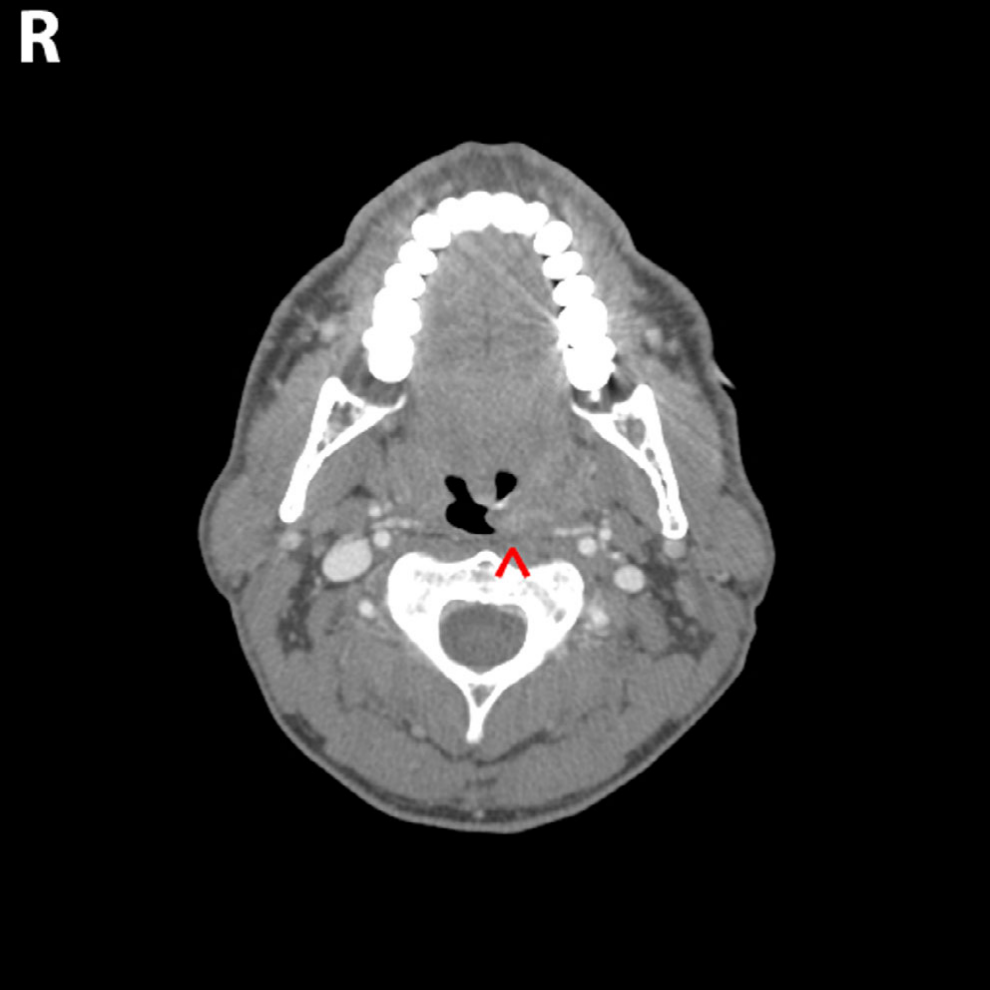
Computed tomographic (CT) neck with contrast. Axial view showing left palatine tonsillar mass extending to midline measuring 2 × 72.5 × 2.7 cm (red arrow)
